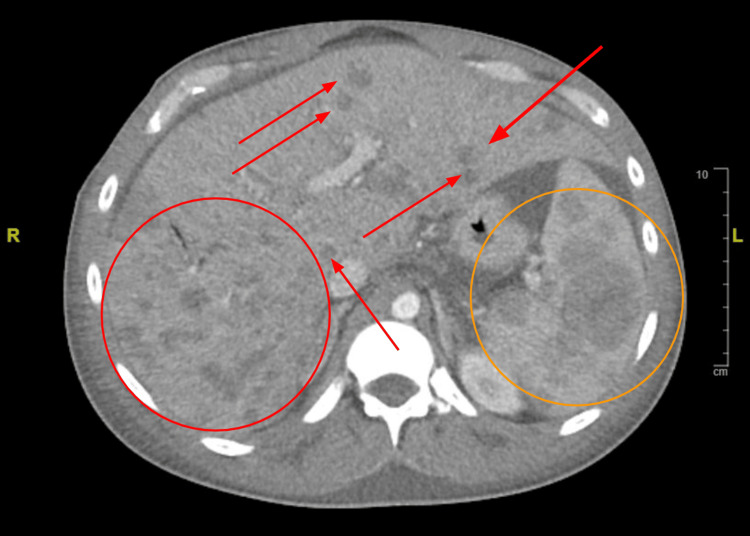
CT chest/abdomen/pelvis with IV contrast (axial view) The image displays multiple hepatic nodules and metastatic disease involving the liver and spleen (spleen seen inside the orange circle, hepatic nodules notated with red circles and arrows) CT: computed tomography
